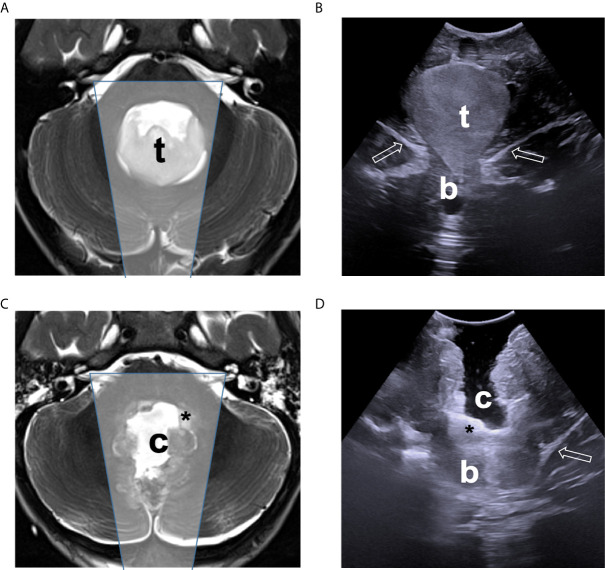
Comparison of ioUS with pre- and post-operative MRI images of a IV ventricle low-grade glioma. Pre-operative (A) and post-operative (C) axial T2-weighted MRI sequence demonstrating the lesion (t), and the surgical cavity (c), tumor residue (asterisk) respectively, with approximate ioUS field of view (shaded box). Intraoperative US view of the same is shown before dural opening (B) and after resection (D), documenting the presence of a small tumor remnant (asterisk) which was intentionally left in place to avoid damage to the IV ventricle floor structures.
